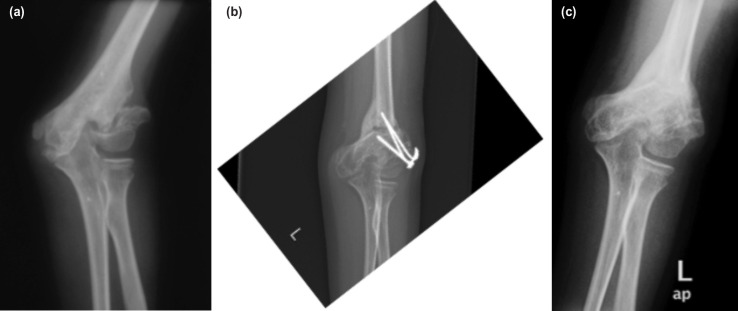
Radiograph of the left elbow. (a) Pre-operative film showing left cubitus valgus with non-union lateral humeral condyle. (b) Post-operative film after corrective osteotomy in the process of healing two months after surgery. (c) Post-removal of implants after three months.
